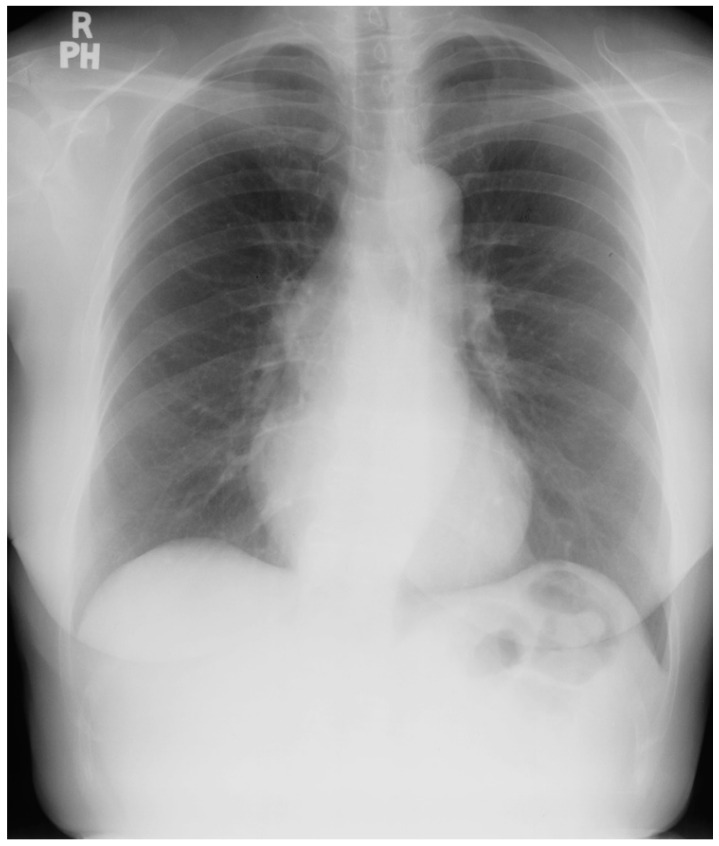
Posteroanterior chest X-ray of persistent left superior vena cava: This chest X-ray was obtained in an elderly female with angina pectoris who was preparing for coronary artery bypass surgery for ischemic heart disease. The image is rotated slightly to the left. There is a good inspiration and the cardiothoracic ratio is normal. The lung fields are clear and the pulmonary vascularity is normal. In this case of persistent left superior vena cava (PLSVC), the ascending aorta is more clearly imaged because the normal right-sided superior vena cava is not present to obscure the ascending aorta. Overlying the upper descending aorta and coursing parallel to the vertebral column and crossing the left main bronchus is a vascular structure that appears to be the PLSVC, prior to draining into the coronary sinus.
